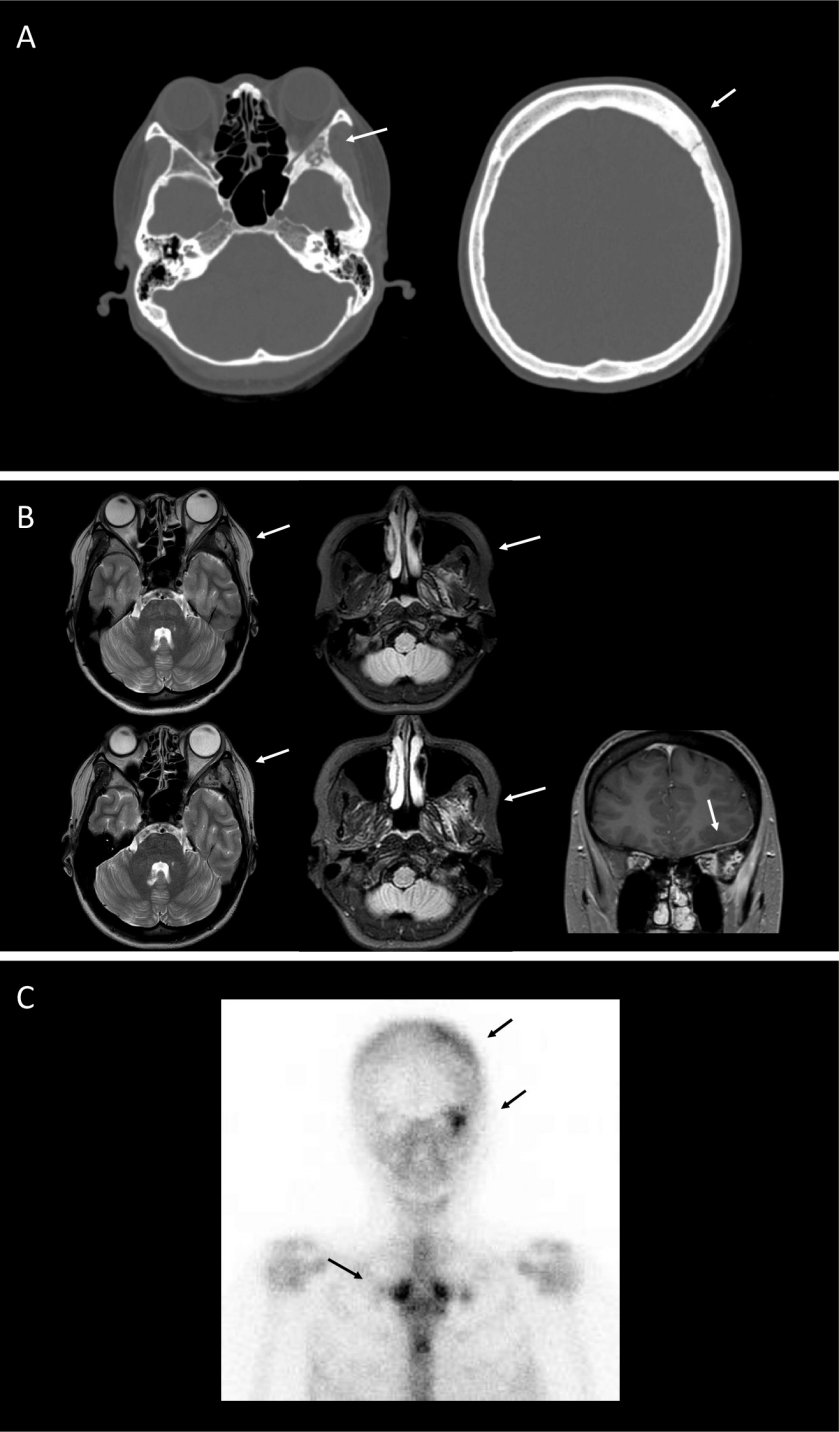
(A) A CT scan of the skull showing osteolytic and osteosclerotic changes in the sphenoidal, frontal and parietal bones on the left side (arrows). (B) An MR scan of the brain: initial (upper row) and current (lower row) presentation. Images show T2 hyperintensity in the sphenoidal bone (upper and lower left, arrow), fluid-attenuated inversion recovery hyperintensity in the temporal and pterygoid muscles (upper right and lower middle, arrow), and thickening and gadolinium enhancement of the dura mater in the left frontal region (lower right, arrow). (C) Bone scintigraphy showing increased uptake in both clavicles, sternum, and sphenoidal and frontal bones on the left side (arrows).
